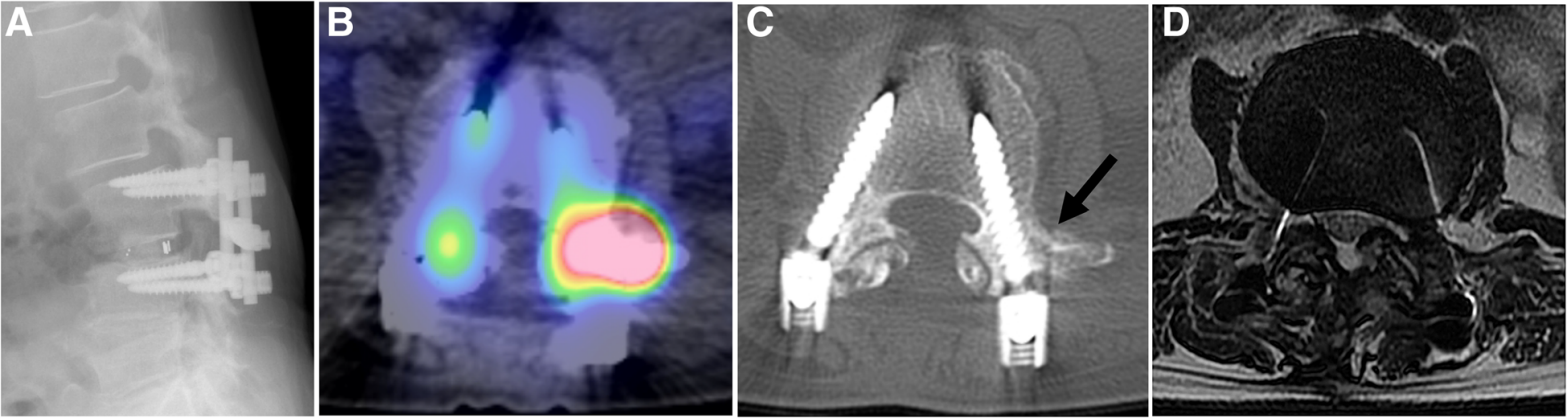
A 67-year-old woman with an insufficiency fracture of the L3 transverse process. a Lateral view of radiograph showing an L2 spondylolisthesis after posterior lumbar interbody fusion of L3/4, with adjacent spinal degenerative disease considered the cause of pain based on pain location and imaging assessment using plain radiographs, computed tomography, and magnetic resonance imaging. b Bone SPECT/CT image showing a hotspot in the right transverse process of L3, and not in the facet joint of L2/3. c On retrospective review of the computed tomography image, the non-traumatic insufficiency fracture of the right transverse process of L3 was identified (black arrow), and the patient was treated using oral steroids for rheumatoid arthritis. d T2-weighted MR axial image without findings indicating the insufficiency fracture of the right transverse process of L3. MR, magnetic resonance; SPECT/CT, bone scintigraphy with single photon emission computed tomography
